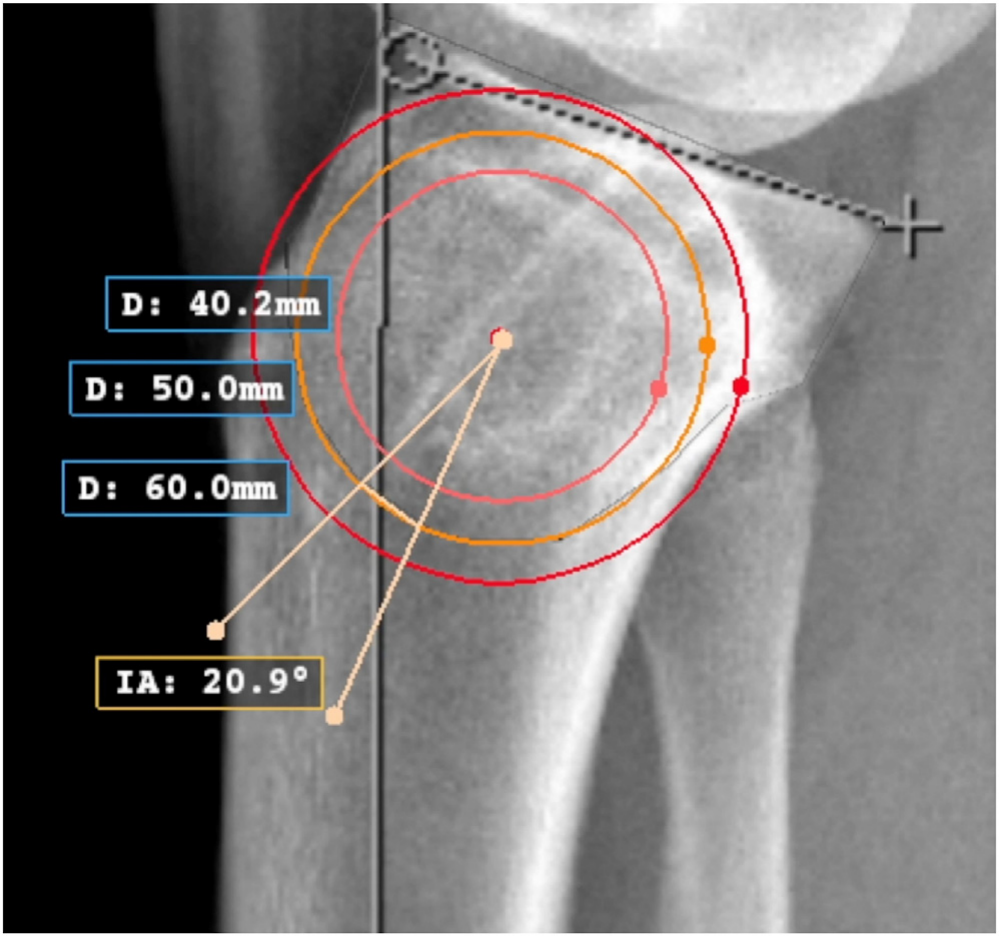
On a lateral radiograph of the right knee, 3 circles centered on the proximal tibial metaphysis are drawn, which represent possible osteotomy curves (red, orange, and pink circles). The radius of each circle should differ by 5 mm to identify what the optimal osteotomy size will be on the basis of unique patient characteristics (D: 40, 50, and 60 mm respectively). The optimal osteotomy curve starts just posterior to the tibial tubercle and ends just anterior to the posterior tibial cortex (orange circle). (D, diameter; IA, posterior tibial slope measure.)
